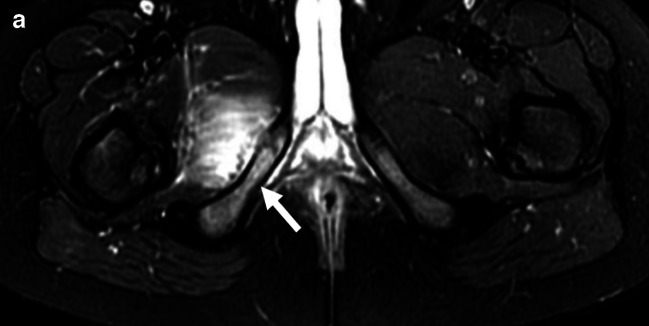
Osteomyelitis at the ischiopubic synchondrosis. A 4-year-old boy presented to the ED with right hip pain, limp, and fever. Initial radiographs (not shown) were unremarkable. a Axial T2W FS MRI demonstrates edema-like signal (*white arrow*) within the bone marrow and soft tissues adjacent to the right ischiopubic synchondrosis. b Axial T1W post-contrast image shows a small subperiosteal abscess (*dashed arrow*)
